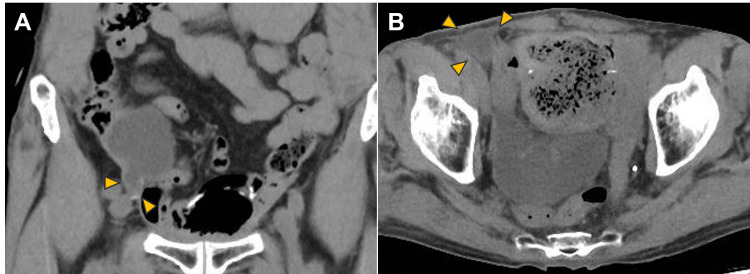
Retrospective review of preoperative CT scans (A) Coronal and (B) axial CT images showing the ileal conduit extending into the right inguinal canal (orange arrowheads). These subtle findings were indicative of incarceration but were initially overlooked due to the rarity of this complication.
